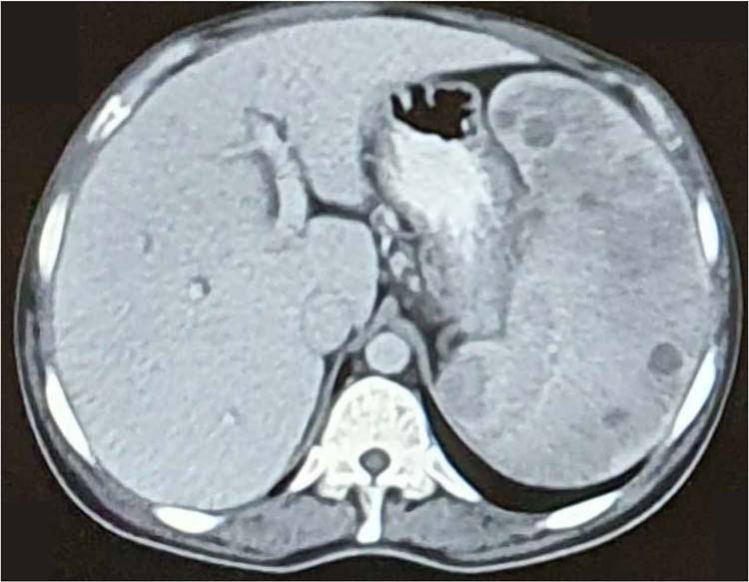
Computed tomography scan of the abdomen shows multiple hypointensities involving the spleen, most likely splenic infarcts, with no evidence of lymphadenopathy.
